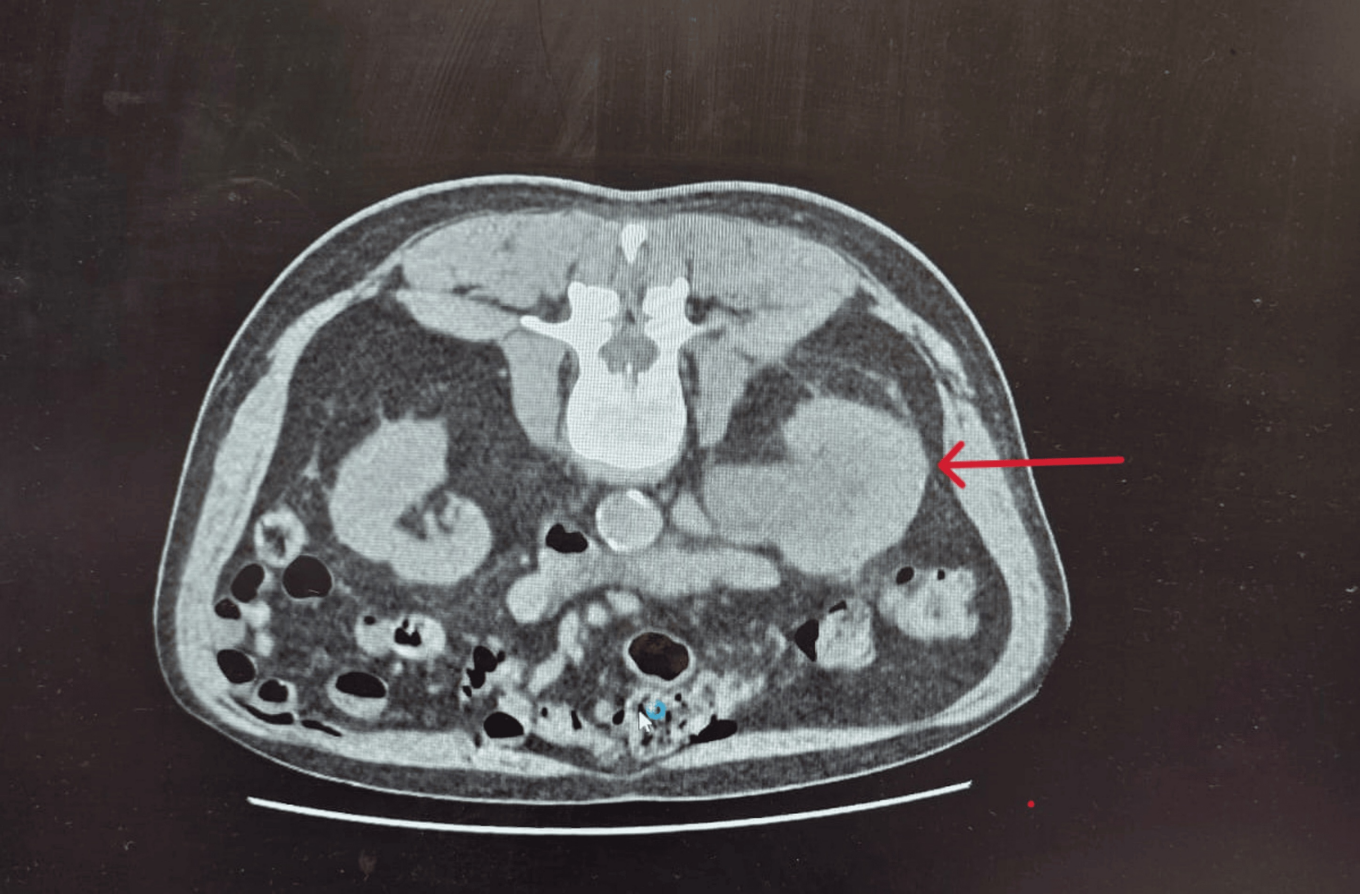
CT kidney-ureter-bladder (KUB) CT kidney-ureter-bladder (KUB) showing right hydronephrosis (red arrow) secondary to obstruction concerning for transitional cell carcinoma.
